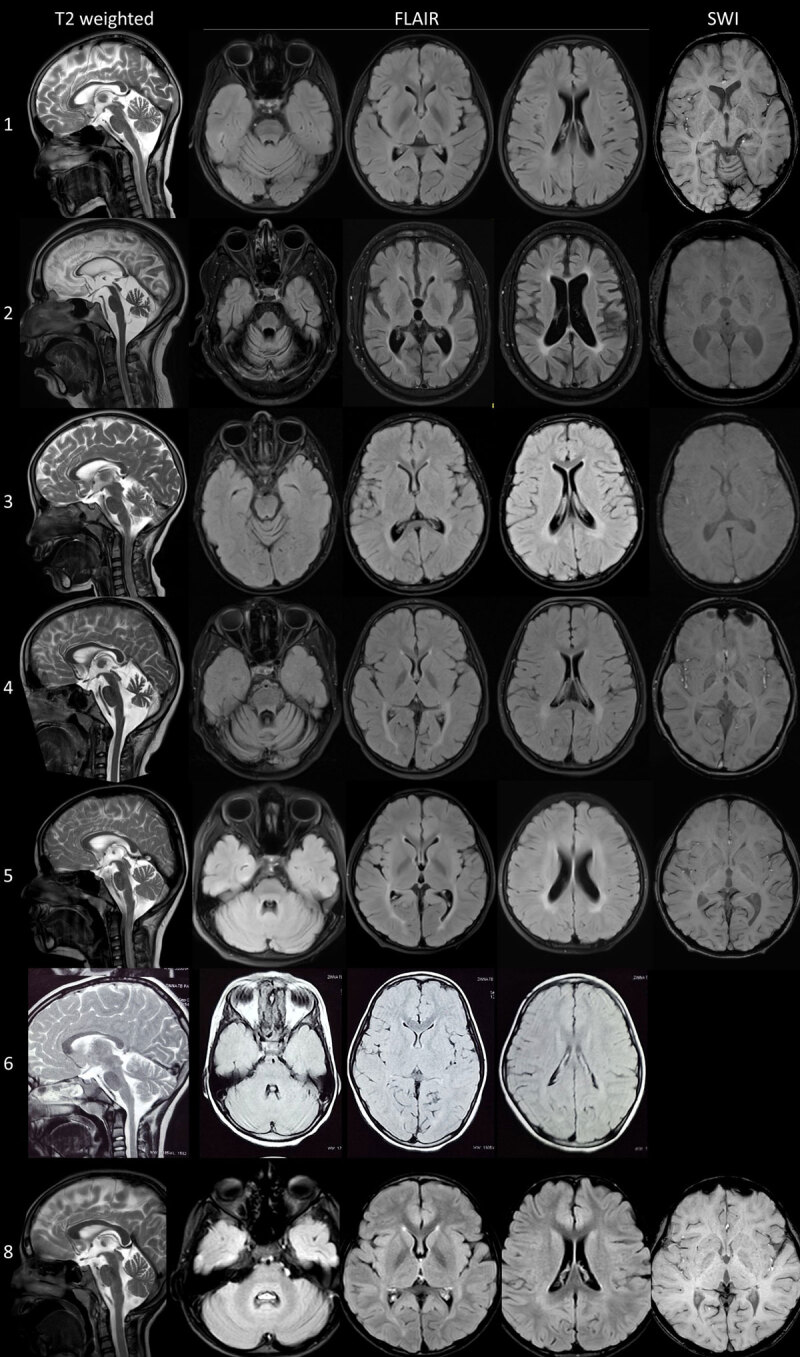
Magnetic resonance imaging of the cohort. T2, FLAIR, and SWI sequences of the MRI brain of the patients demonstrating callosal atrophy, cerebellar atrophy, and periventricular white matter hyperintensity in all, GPi hypointensity on T2 sequences in Cases 1, 2, 4, 5, and 8, and on SWI sequences in Cases 1, 2, and 4. Snapshots of the MRI of Case 7 were not available. FLAIR: Fluid attenuated inversion recovery; GPi: Globus pallidi interna; MRI: Magnetic resonance imaging; SWI: Susceptibility weighted imaging.
